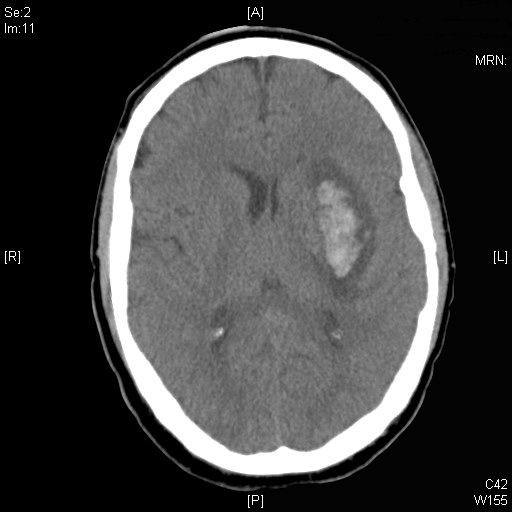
Computed tomography scan of the head demonstrating left internal capsule haemorrhage.
